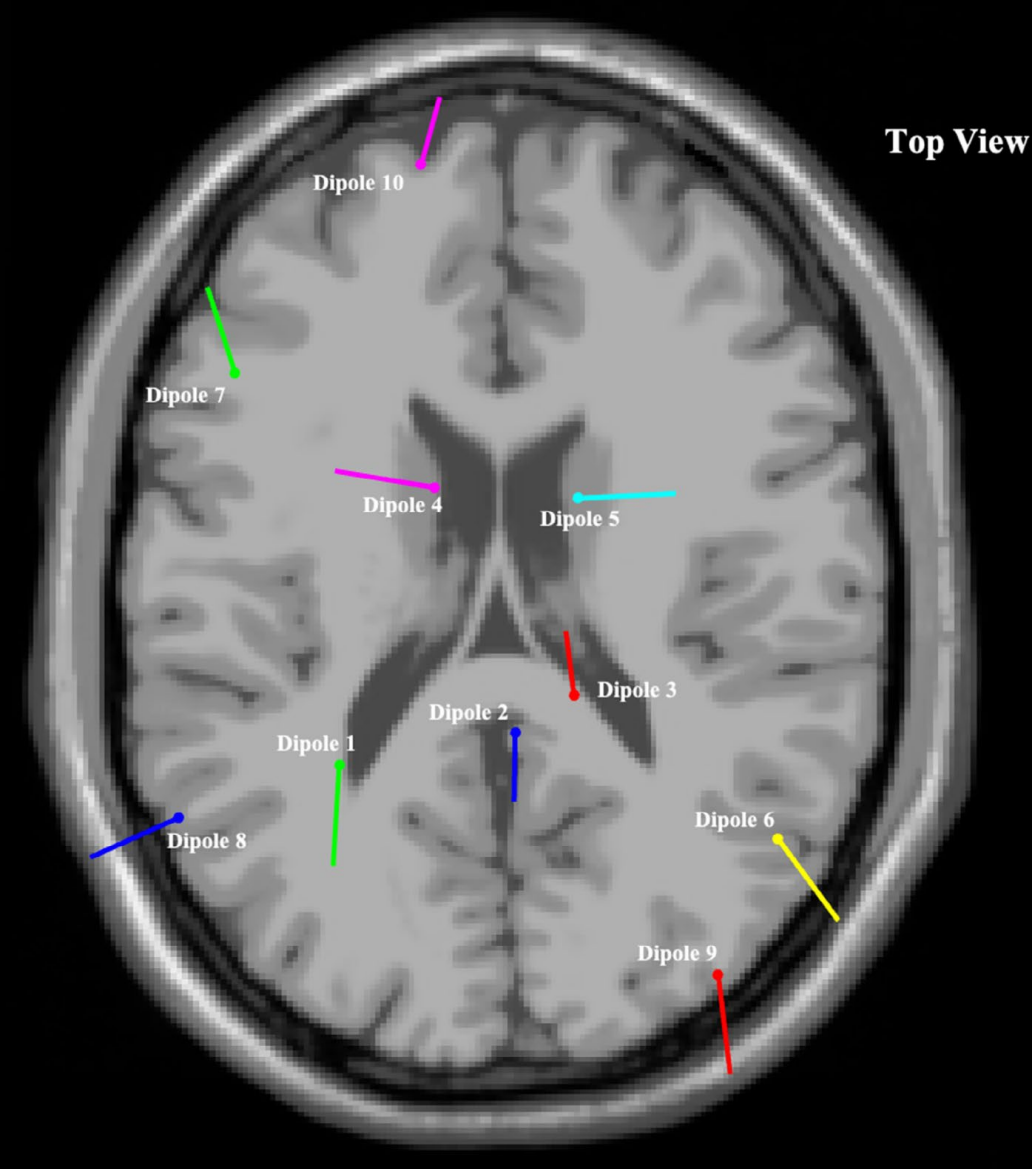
Top view of most significant dipole superimposed on an averaged referenced MRI.
